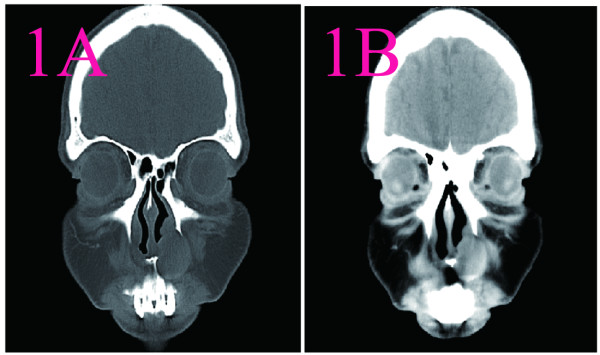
CT scans of nasal cavity in Case 1.A: plain scan: a well-defined ovoid soft tissue mass without central in the right nasal vestibule. The mass of density was uneven(17Hu~43Hu). B: On contrast CT, there was mild enhancement(20Hu~50Hu).
